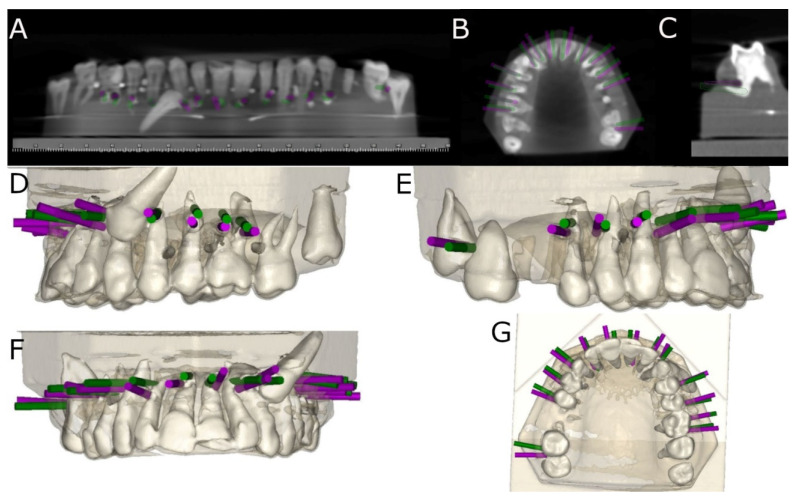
(A) Coronal view, (B) occlusal view, and (C) sagittal view of the CBCT scan of the procedure for analyzing deviations between planned (green cylinder) and performed (pink cylinder) preparations of osteotomy sites. (D) Left lateral, (E) right lateral, (F) frontal, and (G) occlusal view of the postoperatively rendered CBCT scan with the preoperatively planned preparations of osteotomy sites (green cylinders) and the postoperatively performed preparations of osteotomy sites (purple cylinders).
